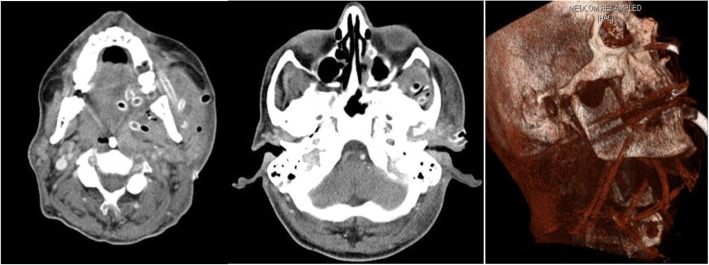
a-b Postoperative CT series in planar depiction after drainage with drains at lingual and buccal sides of the mandible. c CT 3D reconstruction after drainage with 9 tubes All areas with imaging correlated signs
